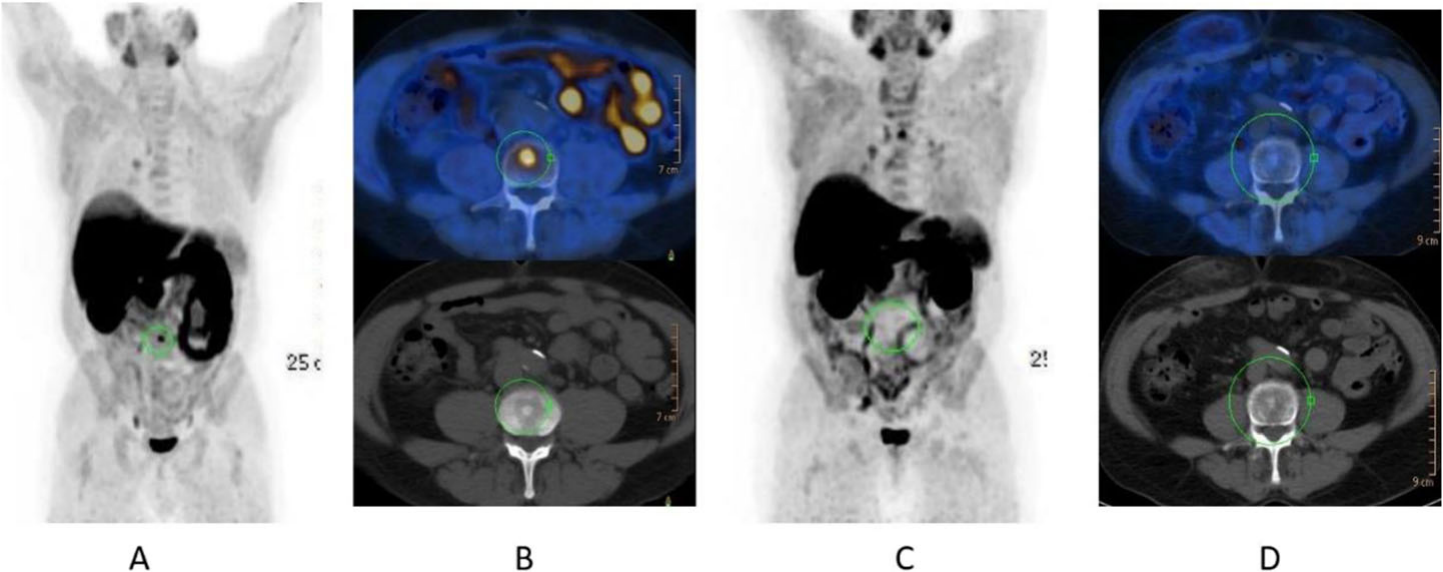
^18^F-fluorocholine, prostate cancer, response evaluation. *Clinical history*: 73 y.o. man with prostate cancer, Gleason score 4 + 3, treated with radical prostatectomy. BCR with PSA 2.3 ng/ml PSAdt 6 months; evaluation after 2 months of hormonal treatment (PSA 0.5 ng/ml). *PET/CT findings*: intense ^18^F-Choline uptake in the vertebra before therapy (a MIP; b fused and CT) not visible after 2 months of hormonal treatment (c MIP; d fused and CT)
